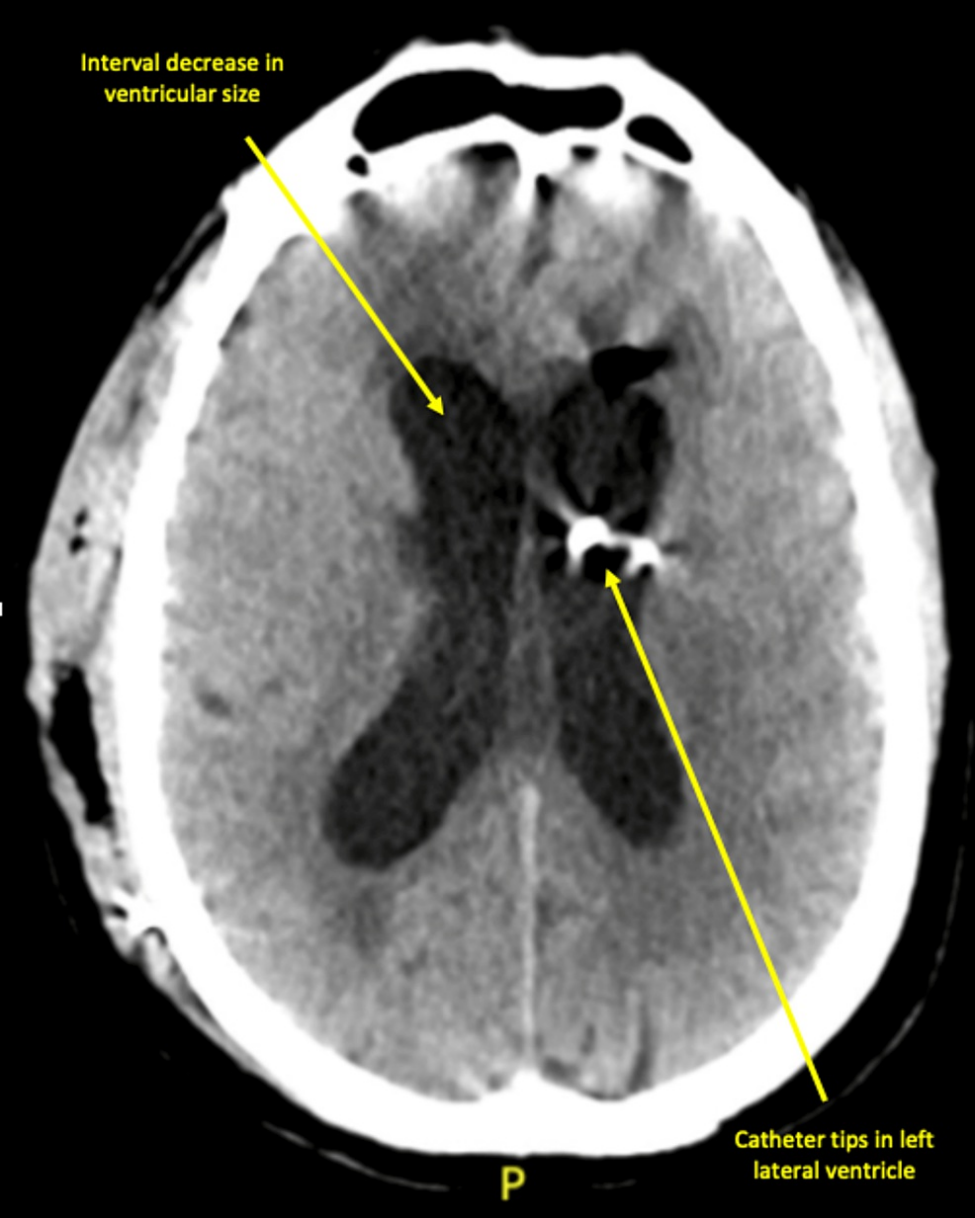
CT head without contrast on day 19 of admission revealed an interval decrease in ventricular size and catheter tips in the left lateral ventricle. “P” shown in the figure represents the posterior aspect of the head.
